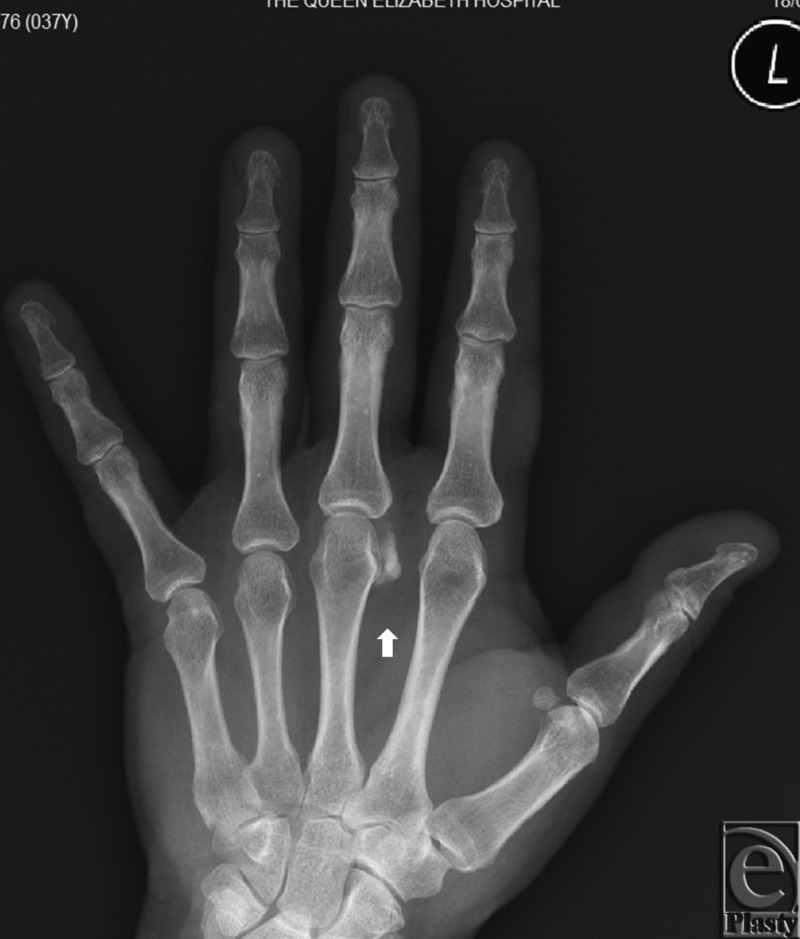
X-ray. Single 12 mm × 4 mm radio-opaque mass (arrow) can be visualized alongside the radial border of the affected joint.
